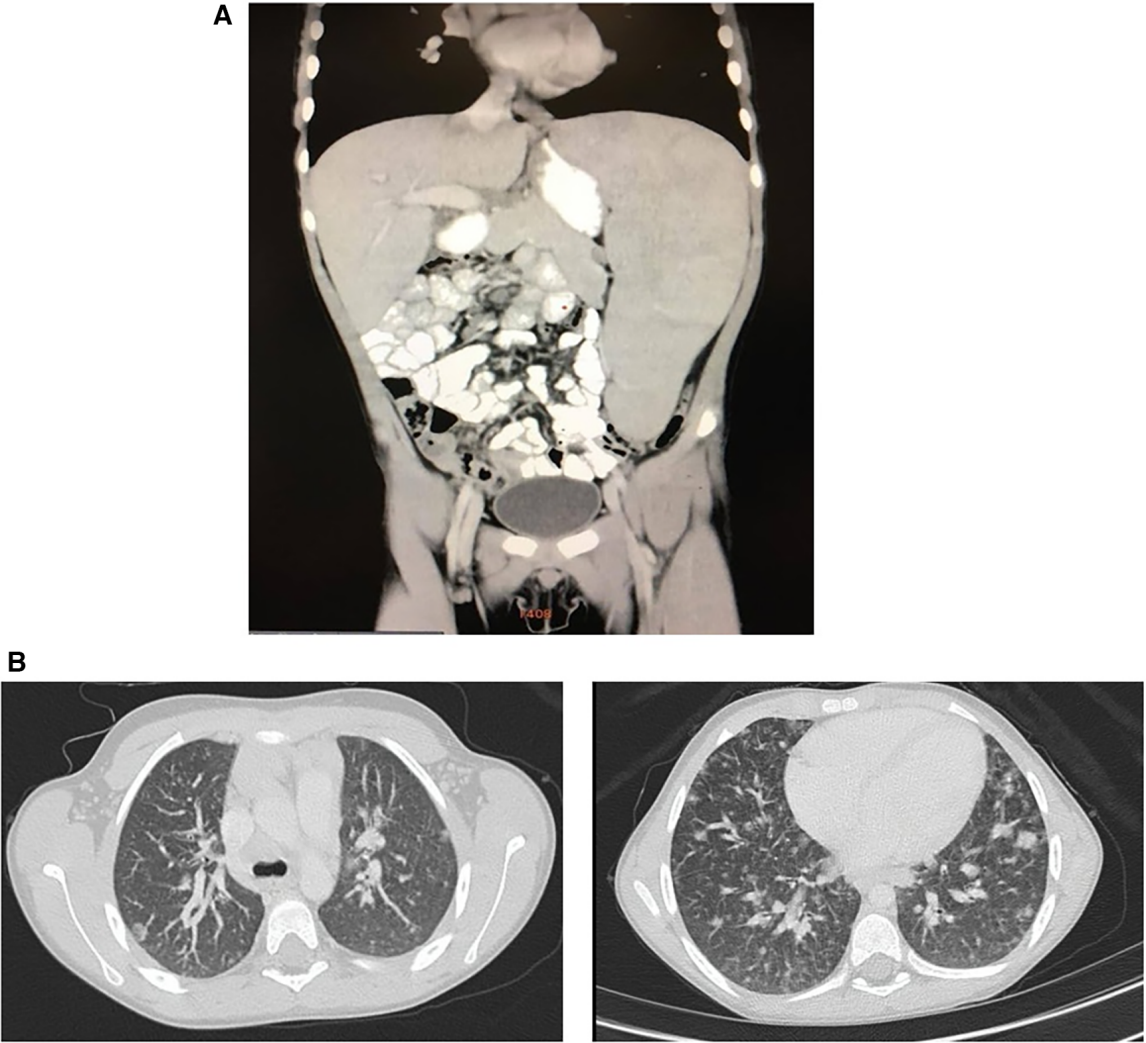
(A) Patient abdominal CT scan. Homogeneous craniocaudal splenomegaly measuring 176 mm associated with increased diameter of splenic vascular structures and increased hepatic hilar vasculature. No evidence of free fluid. (B) Patient chest CT scan. Bilateral diffusely distributed nodules, including solid, subsolid, and ground-glass opacities, predominantly in the lung bases with the largest measuring 12 mm.
